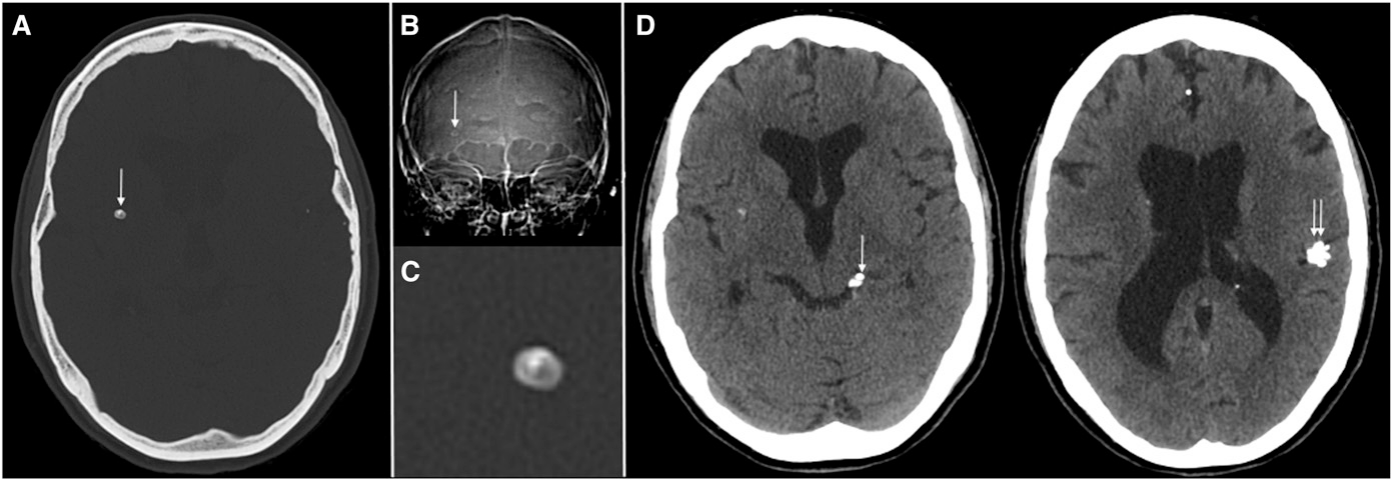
(A) Axial CT scan image (July 20, 2017) in bone windows, showing right basal ganglia calcific lesion with typical outline of a cyst with scolex. (B) Scout view of the CT scan showing the cyst with scolex (white arrow). (C) Magnified view of the cyst on CT. (D) Soft tissue windows of the brain showing multiple other calcific lesions, mainly in the left perimesencephalic cistern (white arrow) and left Sylvian fissure (double white arrows). Smaller calcifications are seen in the anterior interhemispheric fissure and in the left lateral ventricle.
